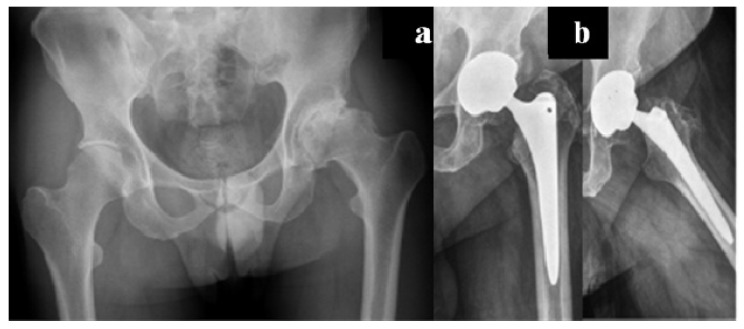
(a) Preoperative X-rays of the left hip of a 58-year-old patient affected by severe haemophilia A; (b) Radiographic aspect 6.5 years after surgery: Standard cementless tapered stem with ceramic-on-polyethylene (COP) coupling.
